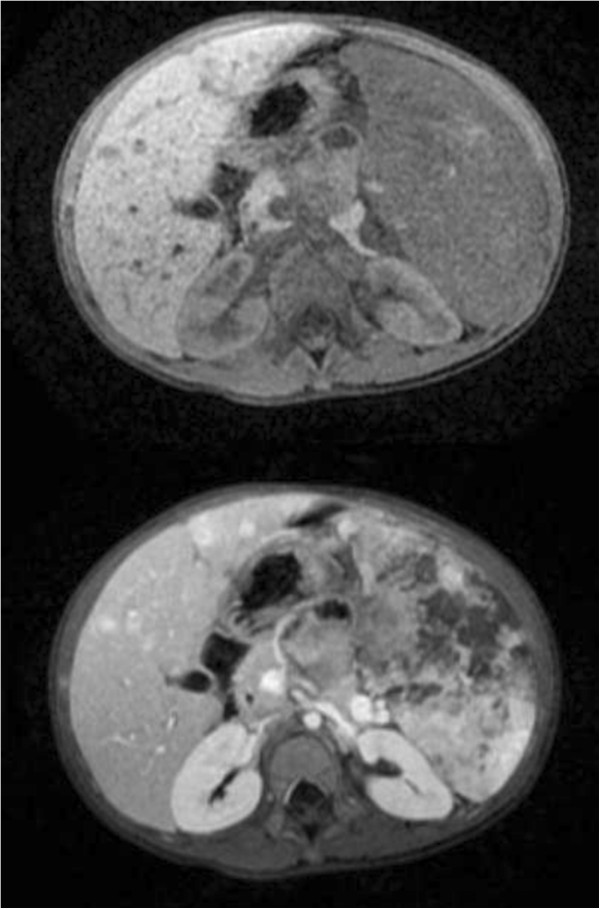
Axial pre- and post-contrast T1-weighted magnetic resonance images. The pre-contrast T1-weighted image demonstrates an enlarged spleen with a small area of high signal in the periphery, suggestive of focal hemorrhage. Pre-contrast T1-weighted images also demonstrate three small hypointense hepatic lesions in both lobes of the liver, which show avid enhancement following administration of intravenous contrast. Bottom. Post-contrast T1-weighted images of the spleen demonstrate nearly complete replacement of the spleen by the tumor and by multiple areas of necrosis.
